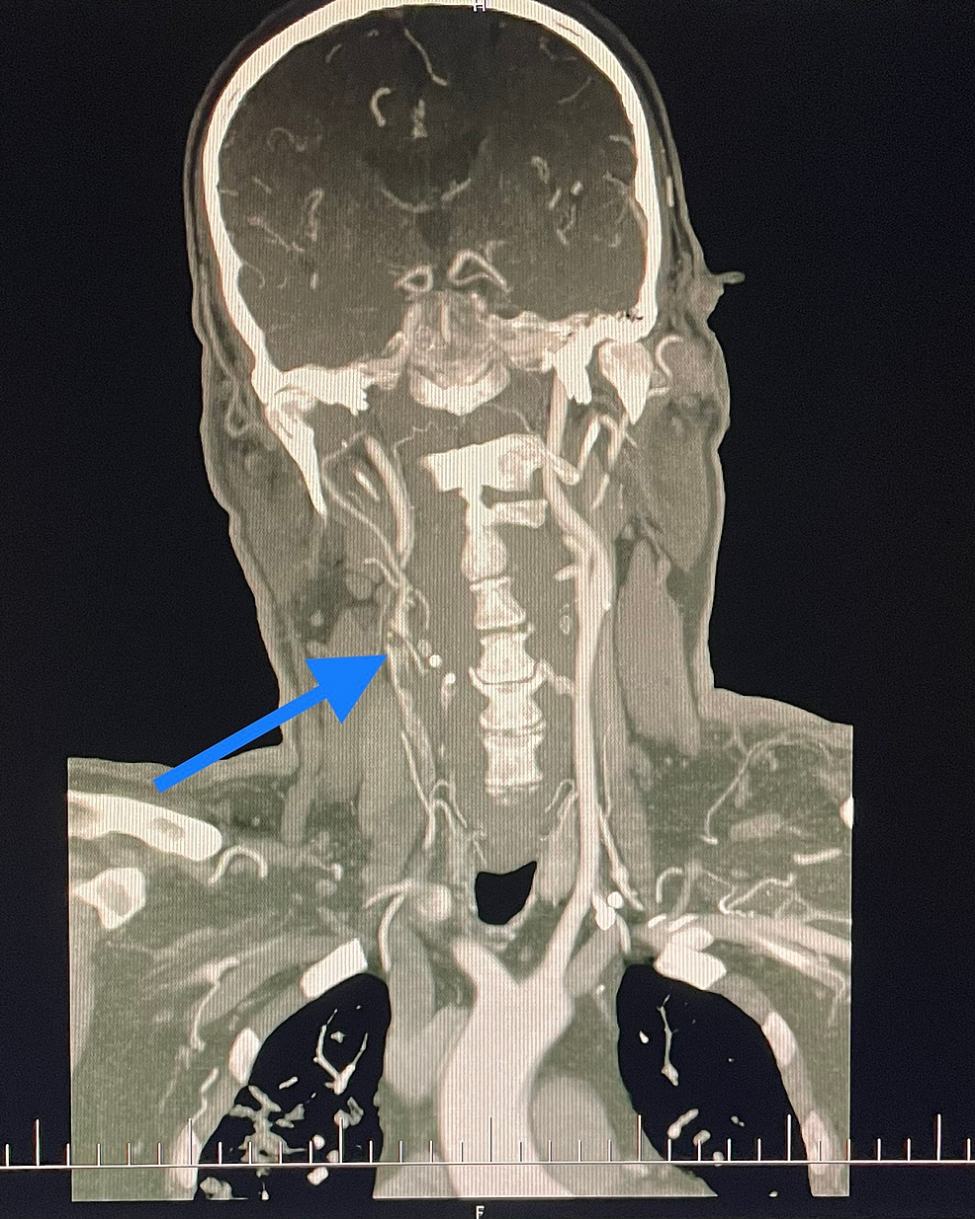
Coronal view computed tomography angiogram (CTA) image shows thrombus in right common carotid artery extending into carotid bifurcation
